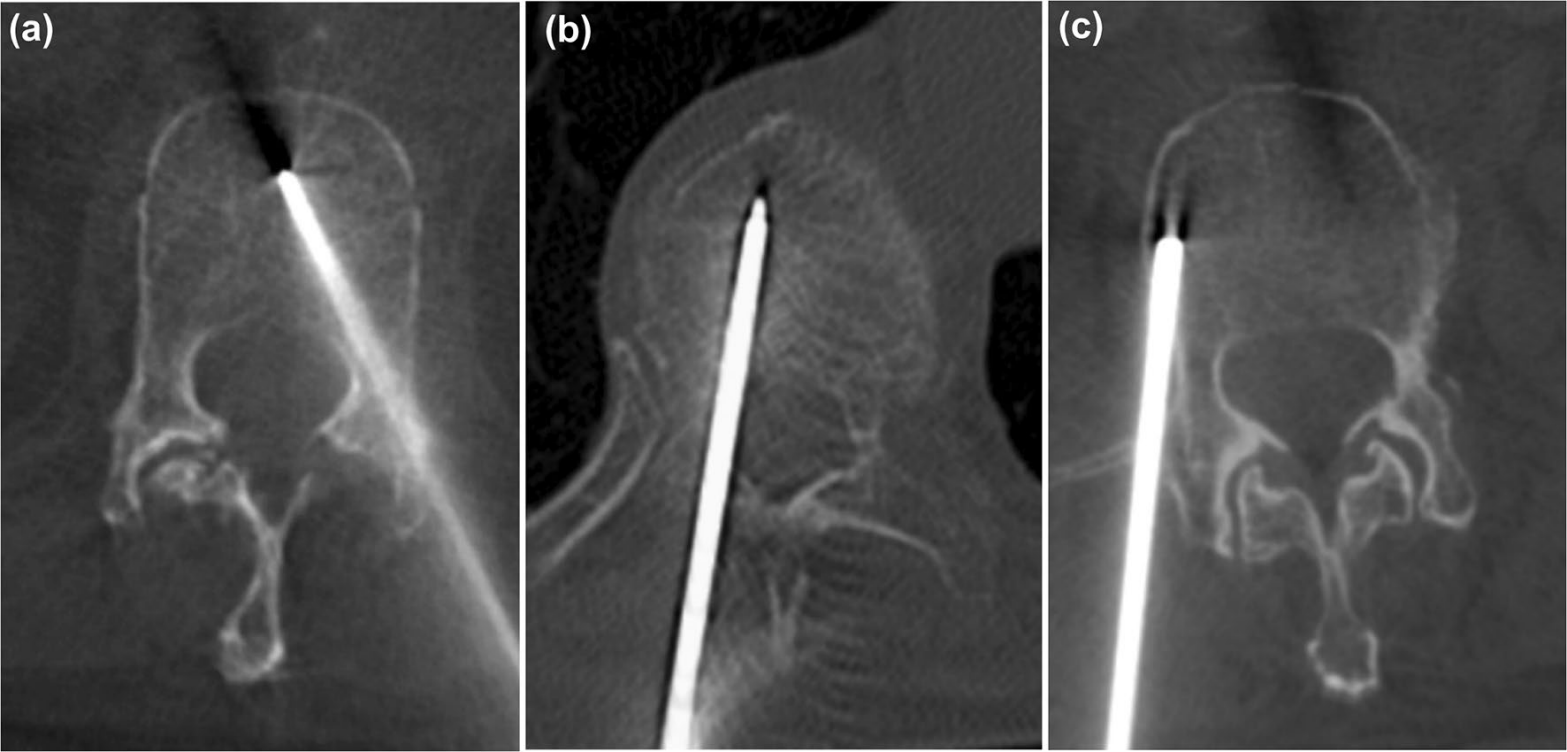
An IVR-CT image (a) and Cone-beam CT images (b and c) of the vertebral body after the bone biopsy needle insertion. Cases a, b, and c are Grade A (no cortical breach) in medial breaches, Grade B (the cortical breach with 2 mm or less) in medial breaches, and Grade C (the cortical breach with 4 mm or less) in lateral breaches, respectively, according to Gertzbein and Robbins classification scores based on CT commonly used to assess the pedicle screw placement in spinal surgery
